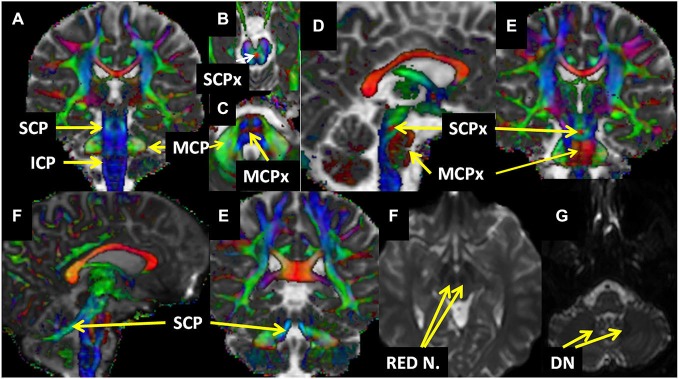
Relevant structures who have been used in the reconstruction are presented on the fused principal eigenvector map (Red-Green-Blue) and MD (A–E) and Diffusion-weighted image (DWI) non-diffusion (F,G) maps. Abbreviations: DN; Dentate Nucleus, ICP: Inferior Cerebellar Peduncle, MCP: Middle Cerebellar Peduncle, MCPx: Middle Cerebellar Peduncle Crossing, Red N.: Red Nuclei, SCP: Superior Cerebellar Pedunles, SCPx: Superior Cerebellar Pedunles Crossing.
